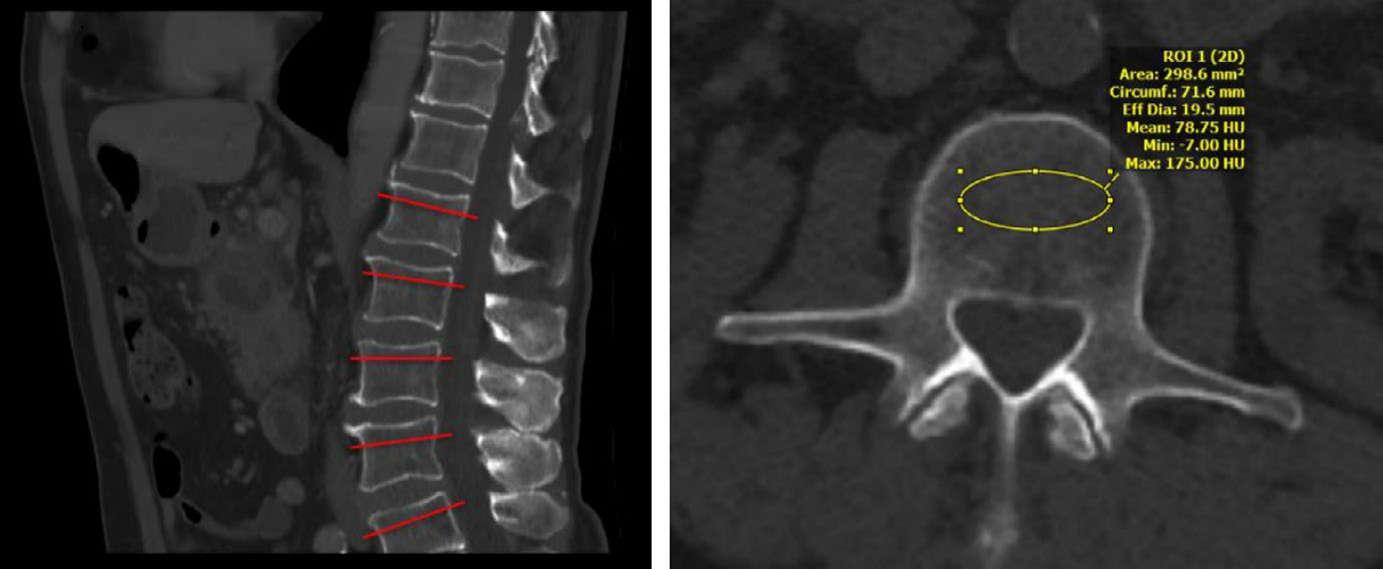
Determination of lumbar vertebral radiodensity. Osteopenia is assessed through routine CT scans using adjusted axial scans that capture the cross‐section of each lumbar (L1–L5) vertebra. Adjusting the scan image accounts for normal shape of the spine and patient position on the scanner bed. Lumbar vertebral radiodensity (LVR and HU) is recorded as the average of the radiation attenuation of the five vertebrae in a region of interest in the cancellous bone of the vertebral body
